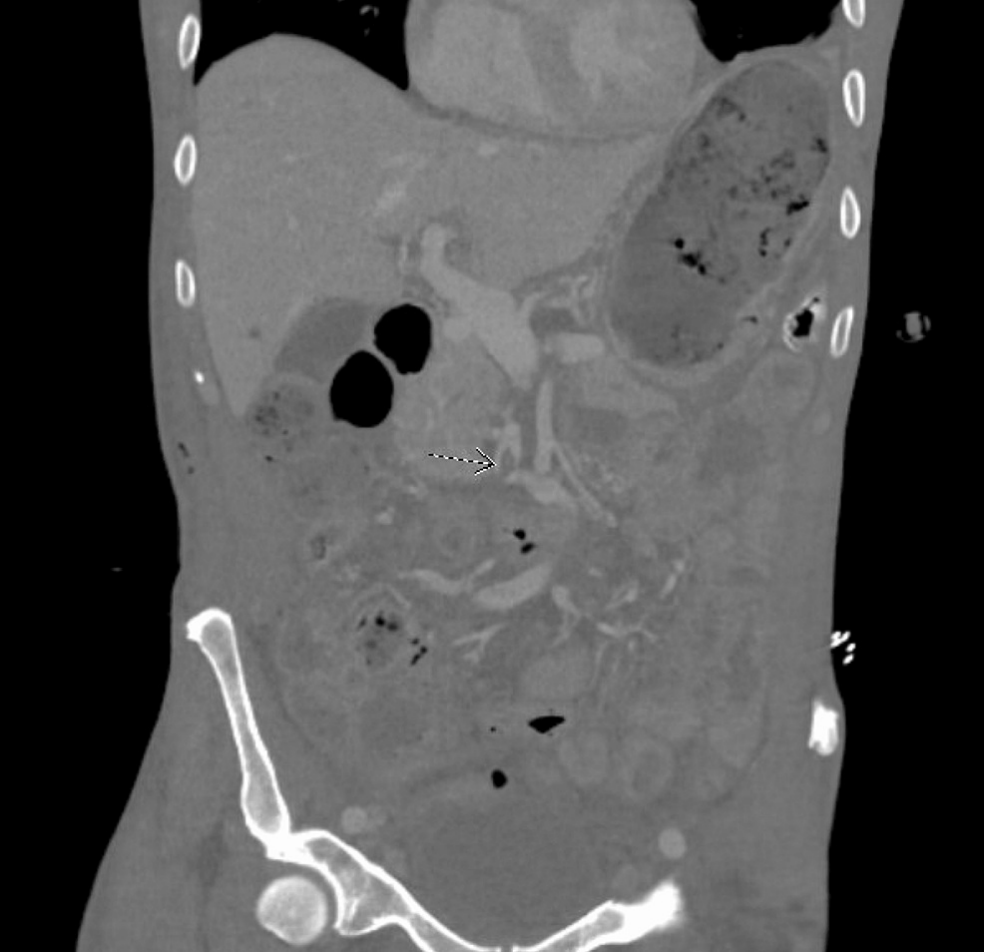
A computed tomography scan of the patient's abdominopelvic area in a coronal view, with an arrow pointing to the site of the superior mesenteric vein thrombosis.
